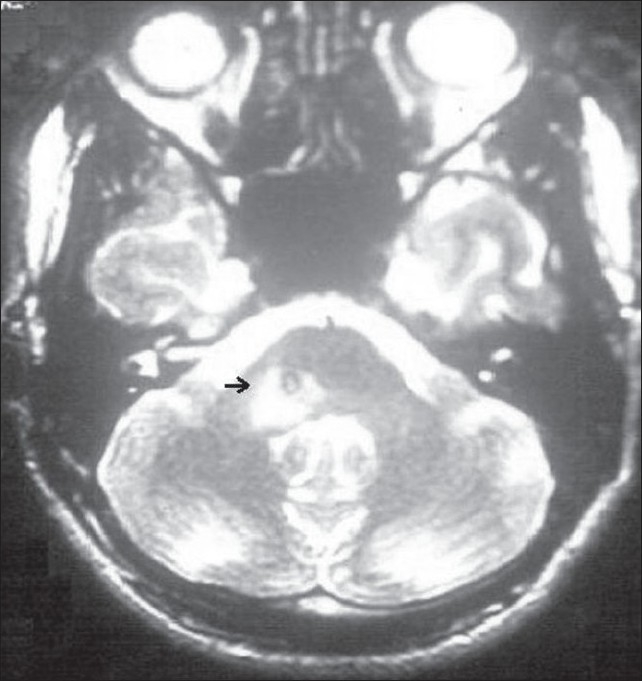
T2 weighted image MRI (axial section) showing an isointense ring lesion with an eccentric hyperintense dot with perilesional edema (arrow)
